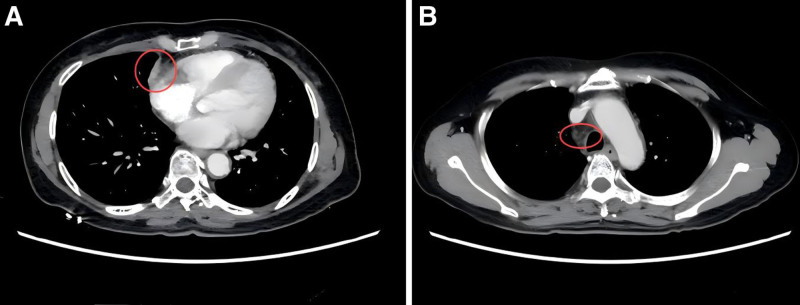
Chest CT follow-up on November 7, 2024. (A) Right lung lesion remains stable; (B) mediastinal metastatic lymph nodes remain largely unchanged in size. CT = computed tomography.
